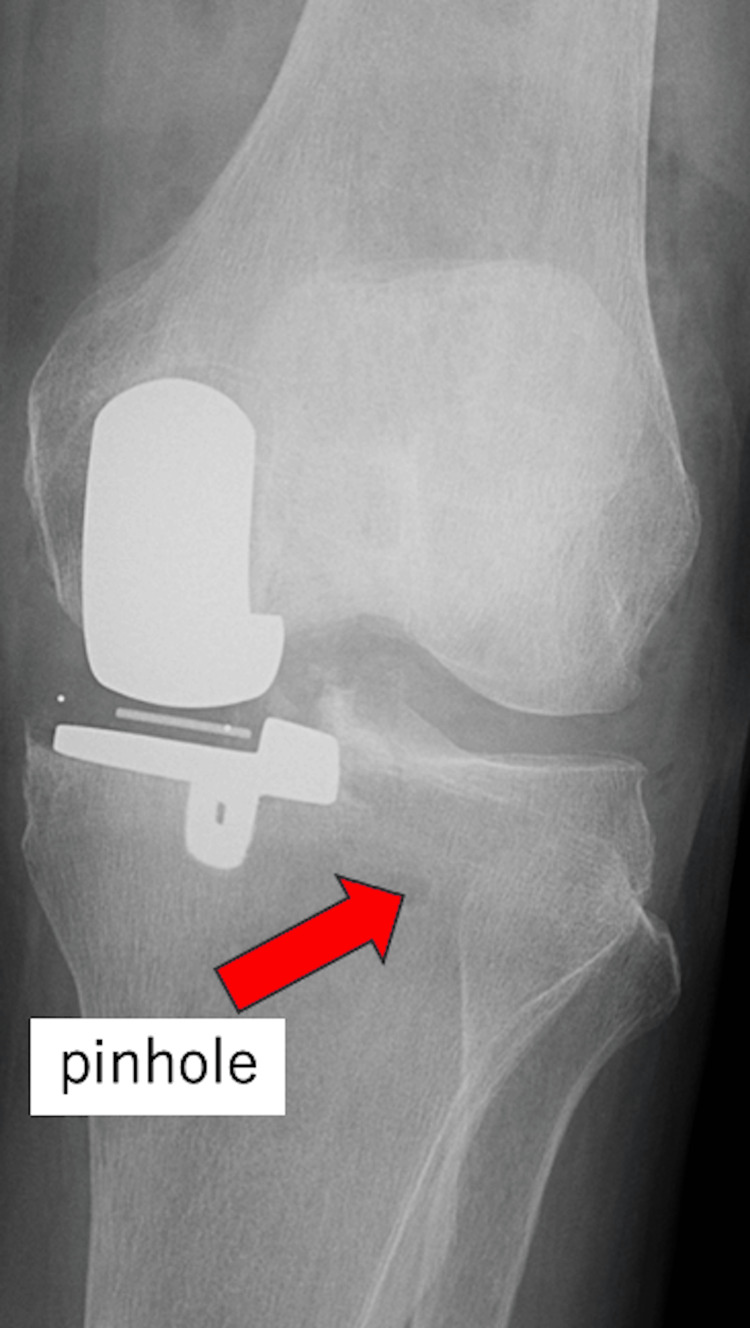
Immediate postoperative X-ray shows no obvious fractures (arrow).
